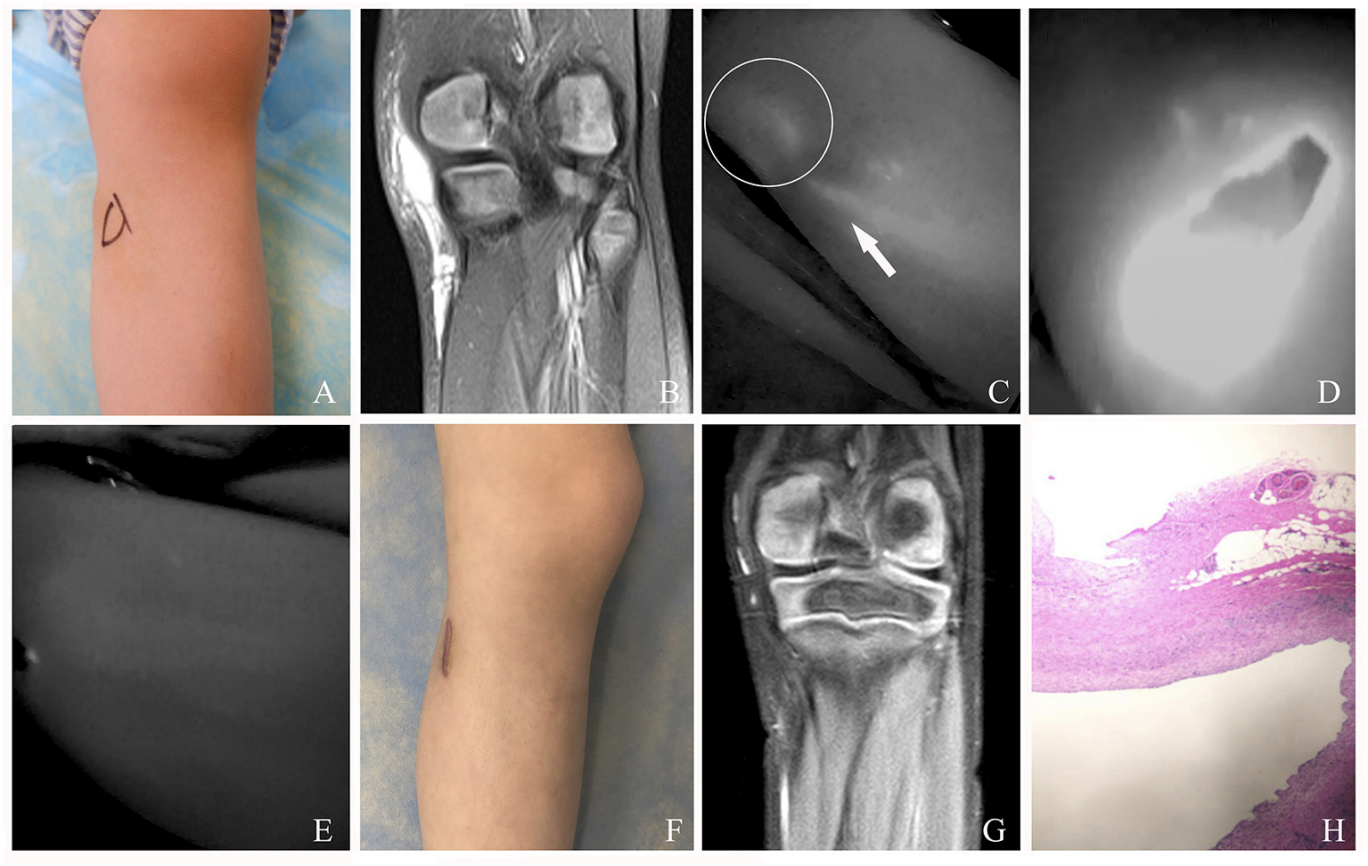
(A) A 5-year-old female patient with mixed cLM of left knee preoperatively. (B) MRI preoperatively. (C) ICG finding with one afferent lymph vessel draining into a lesion. (D) The cLM lesion confirmed by ICG lymphography during surgery. (E) No inflow visualization or lymphatic leak on the previous detected site after inflow occlusion. (F) Twelve months postoperatively, complete regression of cLM met the criterion of excellent curative effect. Remarkably, this patient suffered from skin scar hyperplasia. (G) MRI postoperatively. (H) Pathology postoperatively. *Arrow head*, lymph vessel; *circle*, region of cLM.
